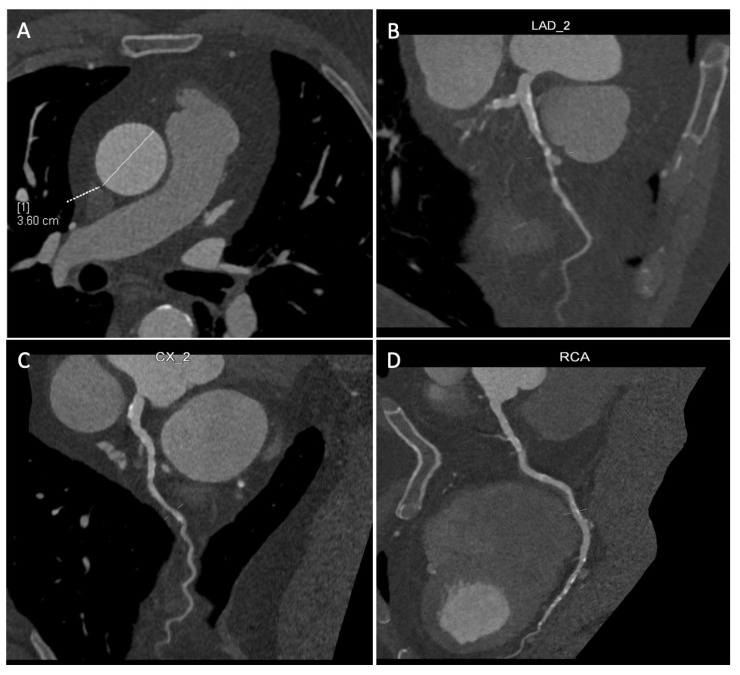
Coronary multislice computed tomography (MSCT) performed before the operation, with the analysis of the ascending aorta (A) and of the three epicardial coronaries (B–D), including the left main. [1] It refers to the calculated diameter of the ascending aorta (white dashed and solid lines).
